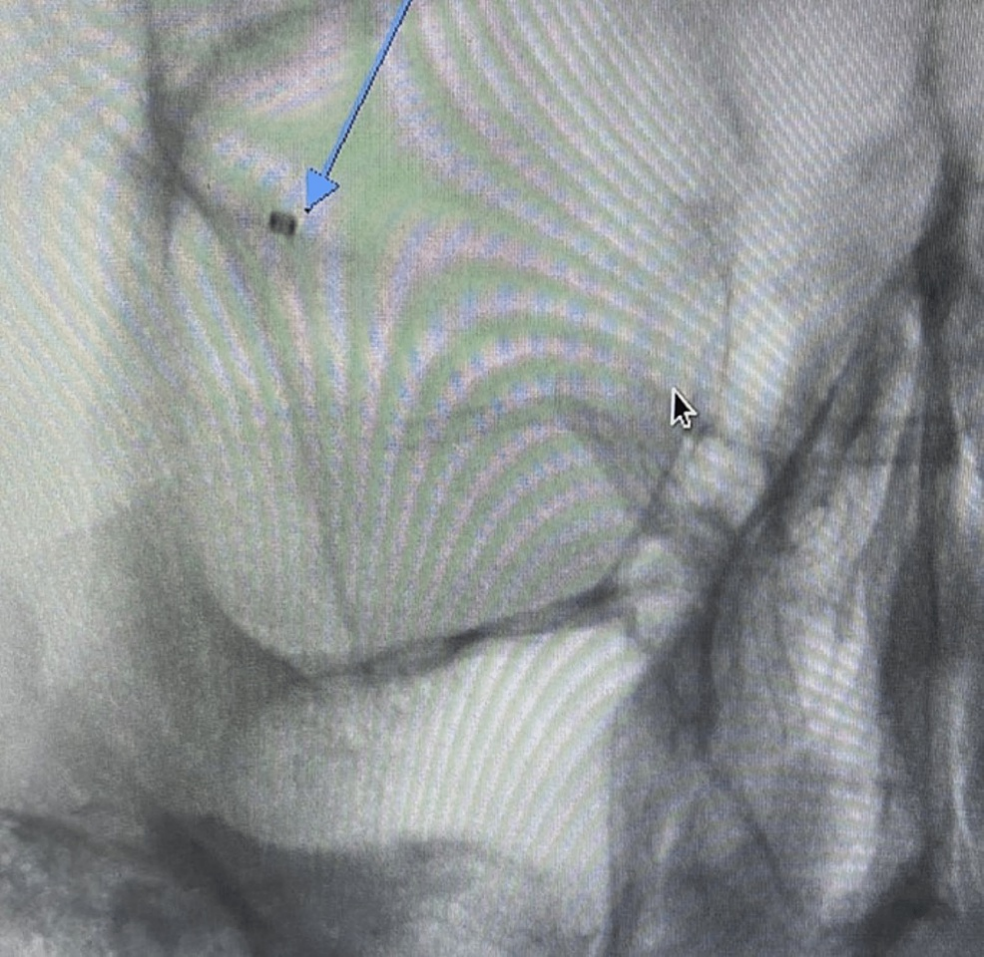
Anteroposterior CT arteriogram showing the aspiration catheter in the occluded M2
